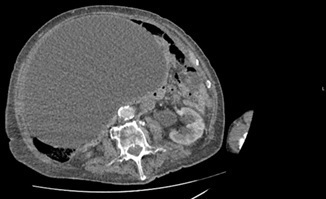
Axial CT image showing a massive homogeneous cystic mass. The wall of this large mass is thin, regular, and smooth and consistent with a fluid-filled mass.
